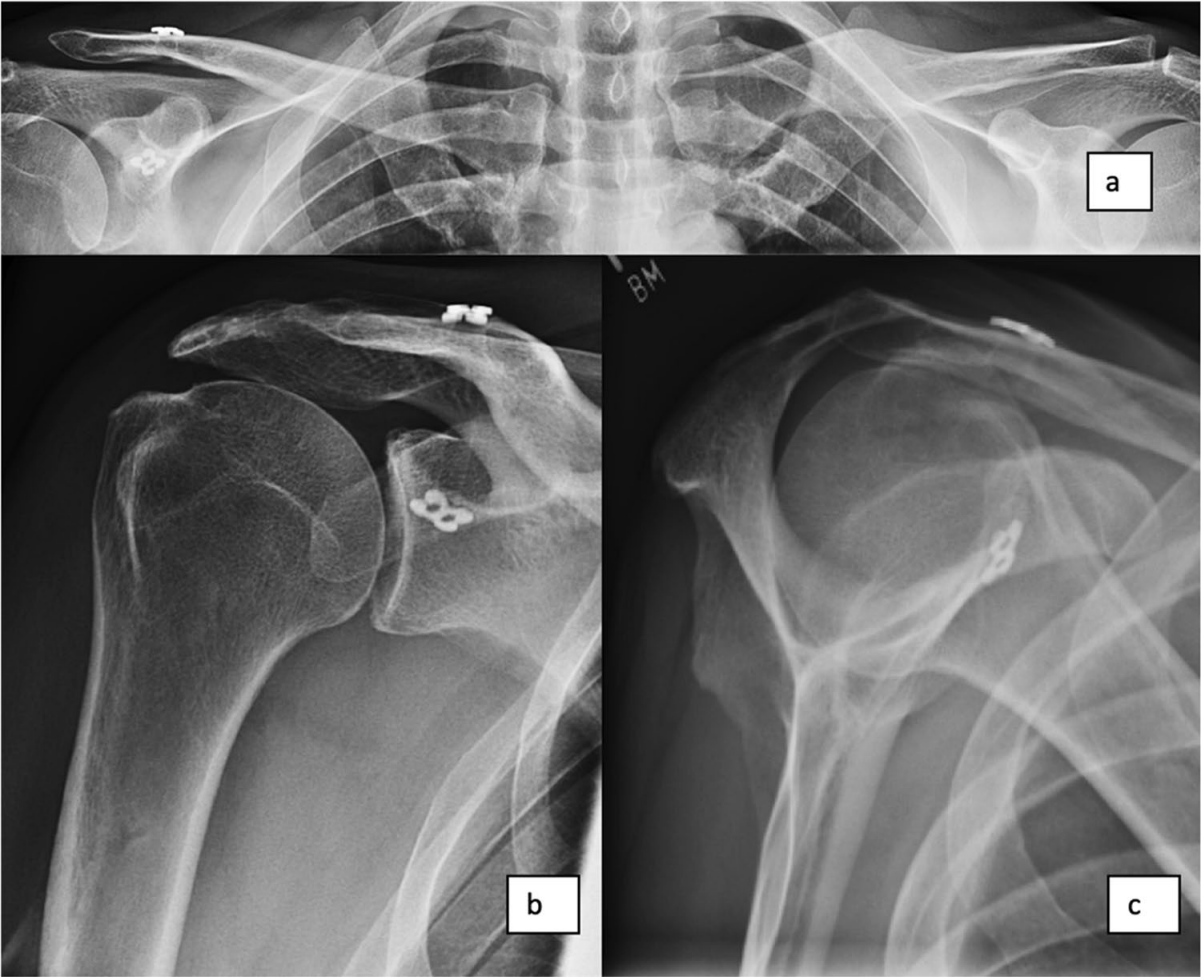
Follow-up X-ray after acromioclavicular (AC) reconstruction with ipsilateral gracilis graft and coracoclavicular (CC) reconstruction with Fibertapes and 2 × Dogbones (Arthrex Inc., Naples, FL, USA); a panorama view, b a.p. view and c Alexander view
